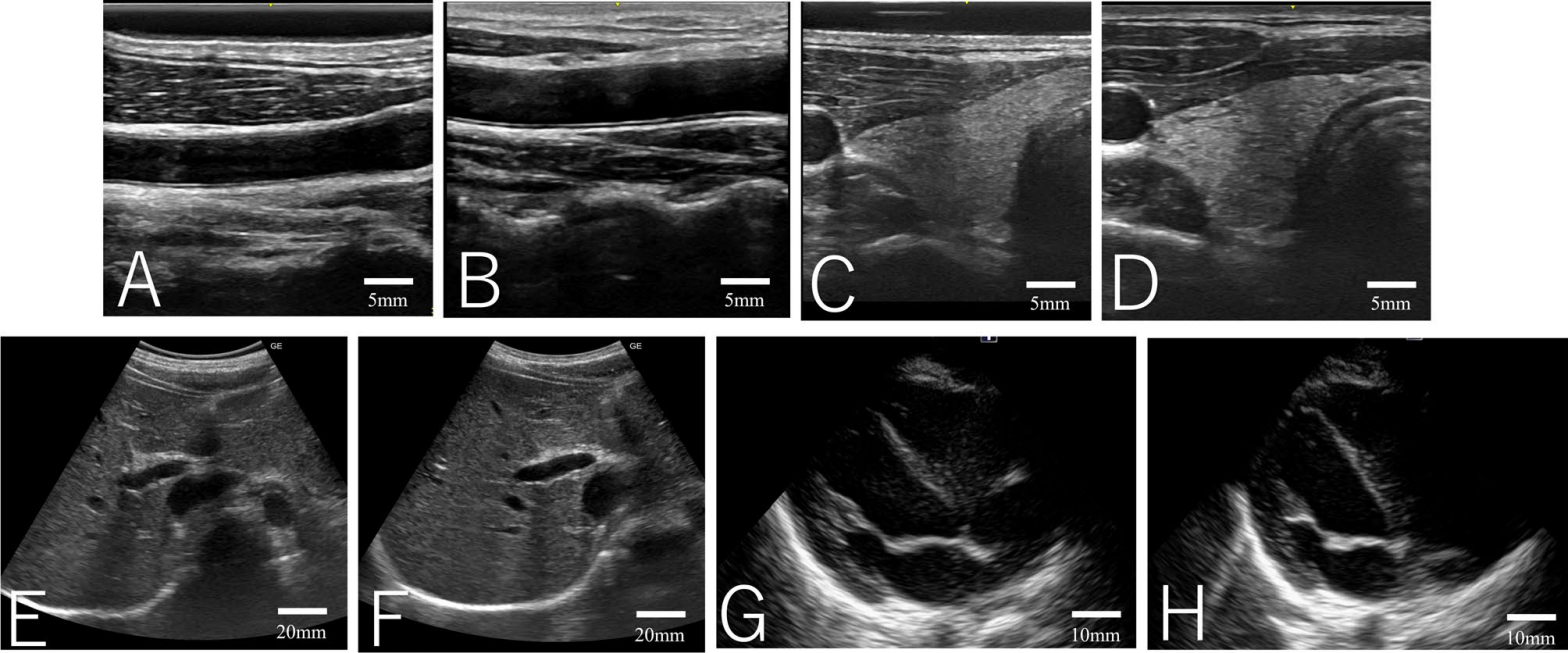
Ultrasound images obtained using our new solid gel and the conventional liquid gel. Common carotid artery: new solid gel (A) and the conventional liquid gel (B). Thyroid gland: new solid gel (C) and the conventional liquid gel (D). Liver: new solid gel (E) and the conventional liquid gel (F). Parasternal four-chamber view: new solid gel (G) and the conventional liquid gel (H).
